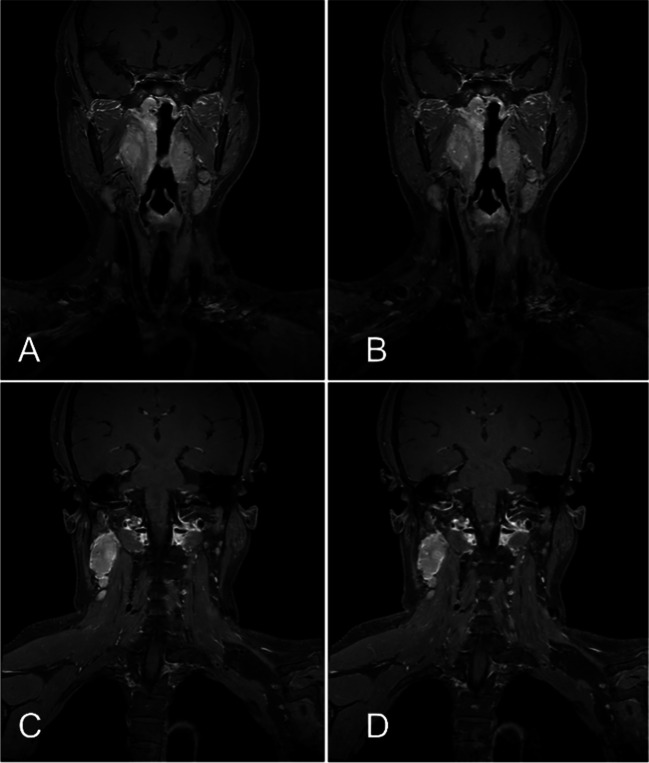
A 54-year-old female patient with NPC. The MRI sequences and examination time were summarized as follows: post-contrast T1-weighted FSE sequences in the coronal planes: (A) and (C) with ACS technique (124 s); (B) and (D) with PI technique (216 s); ACS provided a better image quality than the PI, while the scan time was reduced over 40%
